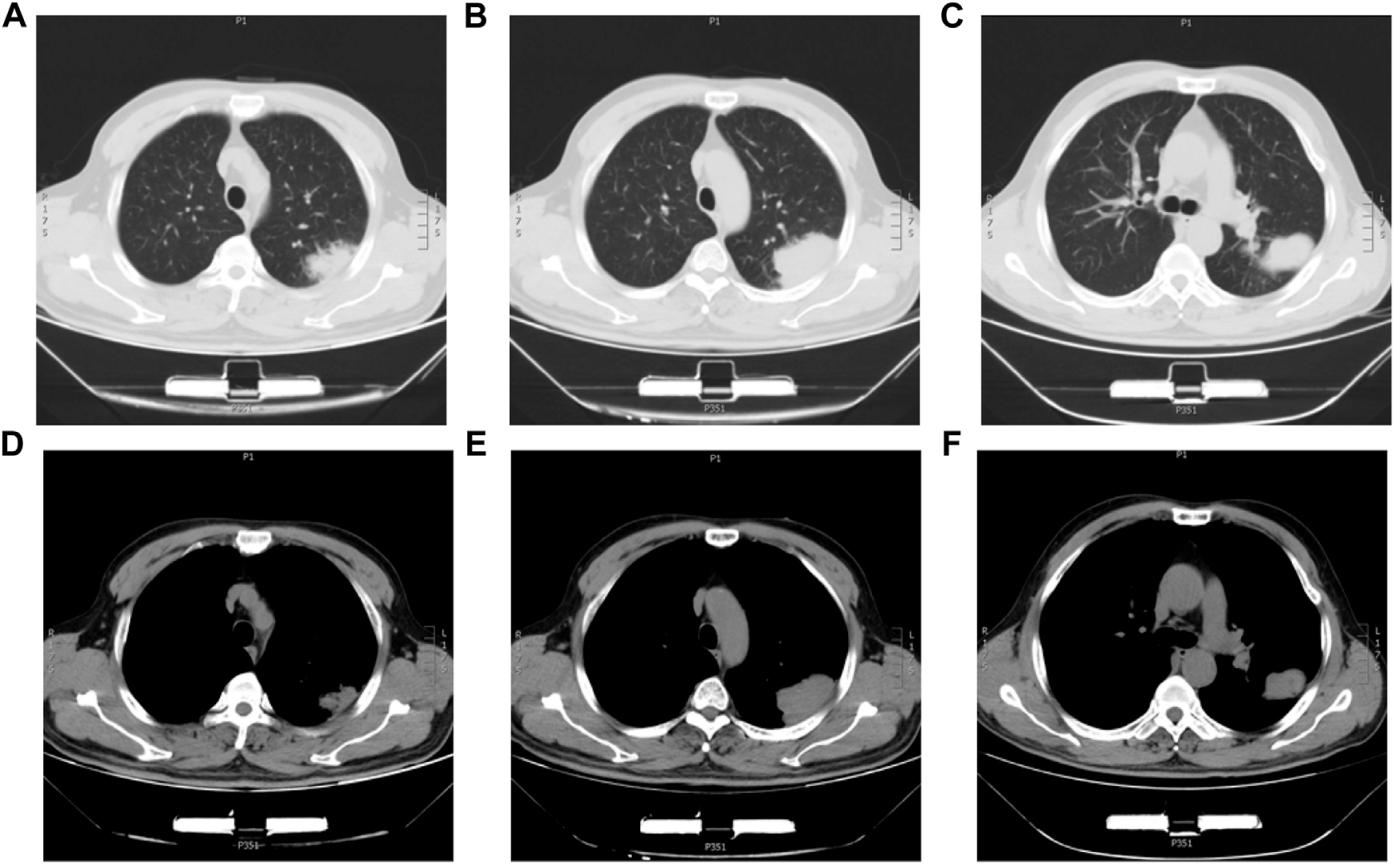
Chest CT images of the HAL patient. (A,B,C) CT images of the lung window with a lung mass in the left upper lobe. (D,E,F) CT images of the mediastinum window with a lung mass in the left upper lobe.
